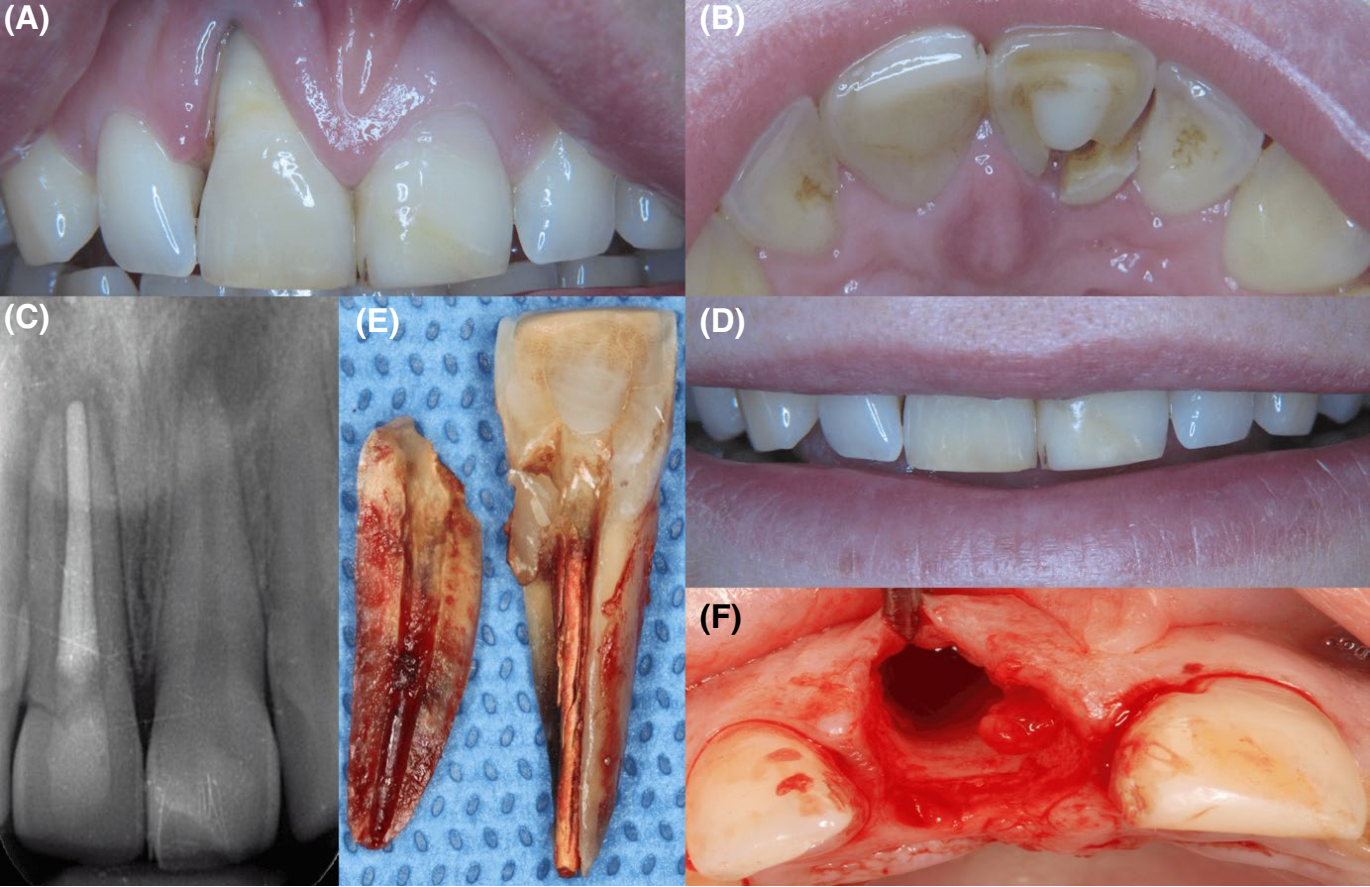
Esthetic and radiographic overview of the failing right upper central incisor. A and B, clearly shows the hard and soft tissue deficiency and the fracture line, respectively. C, shows the radiologic assessment of failing tooth number 11 and fracture line. The patient's smile line was not visibly affected by the tissue deficiency. D, shows the patient's smile line. E, shows the extracted tooth, and F, shows both the hard and soft tissue deficiencies after tooth extraction
